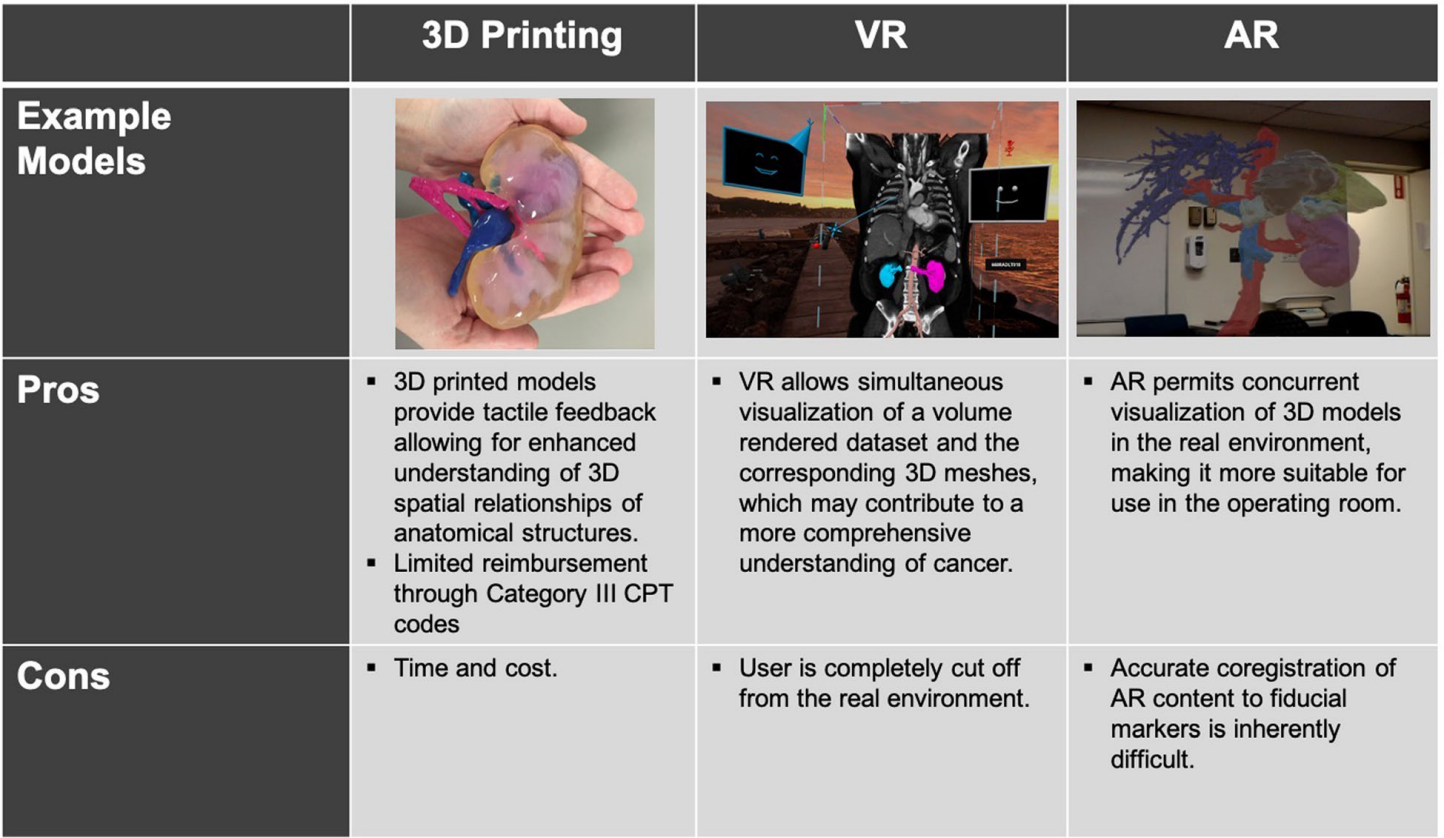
Pros and Cons of 3D Printing, VR, and AR. A multi-colored 3D printed renal cancer model printed with material jetting (Connex 5, Stratasys, Eden Prairie, MN) is shown on the left. Middle: VR visualization of a sagittal CT scan with segmentation of the kidneys (syGlass, Morgantown, WV) viewed through an Oculus Rift headset. Right: AR visualization through the Microsoft HoloLens Device
